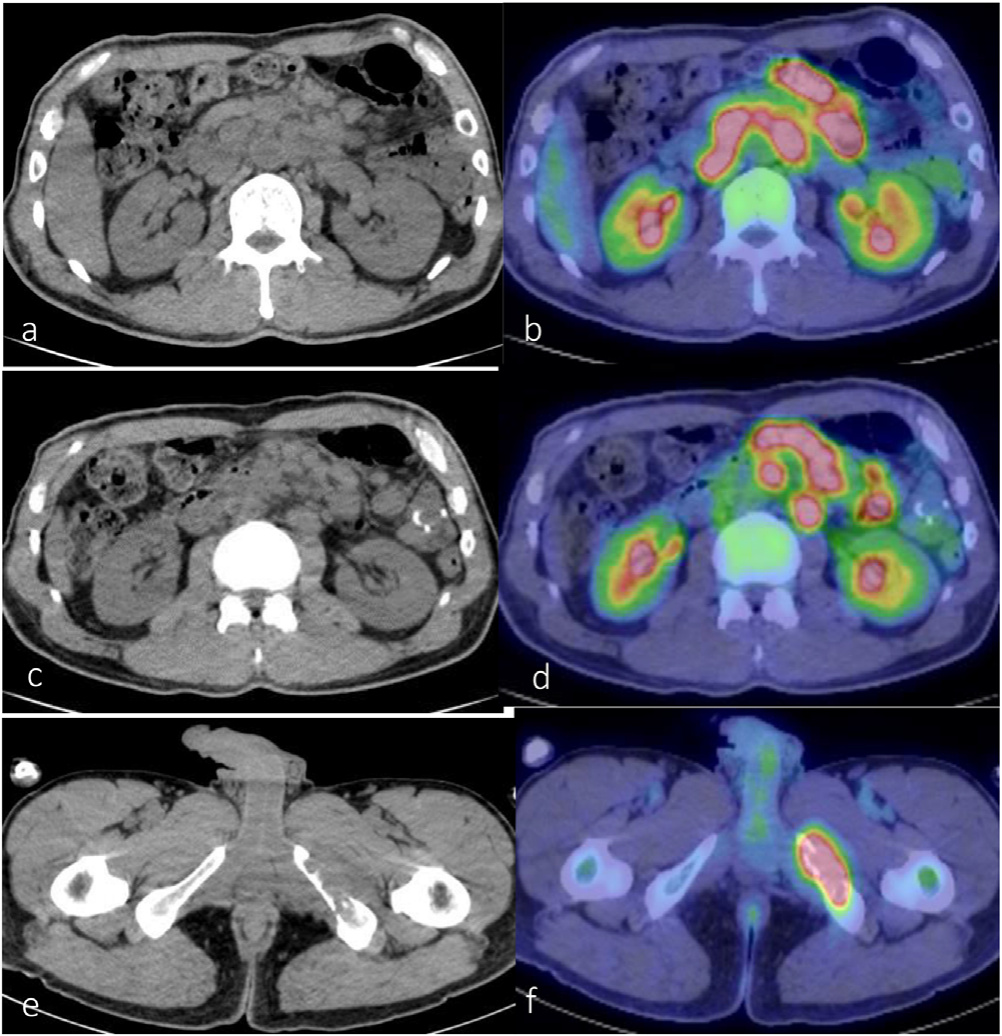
At the initial visit, computed tomography (CT) showed lymph nodes were swelled in heaps around the superior mesenteric artery and the abdominal aorta (a, c). Positron emission tomography / CT showed uptake of F‐18 fluorodeoxyglucose in these lymph nodes (b, d) and in the left ischial bone (e, f).
